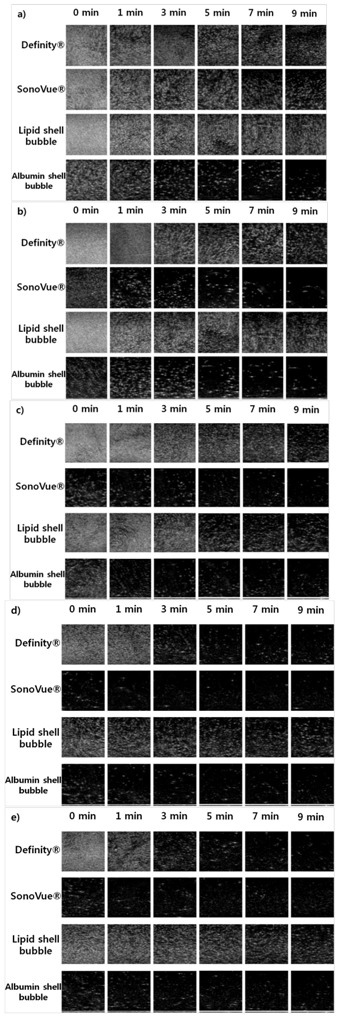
Ultrasound imaging comparison of two commercial UCAs and two synthesized UCAs over time (0, 1, 3, 5, 7, 9 min). (a) 3 days after synthesis. (b) 6 days after synthesis. (c) 9 days after synthesis. (d) 12 days after synthesis. (e) 15 days after synthesis. Ultrasound imaging results show that the synthesized microbubbles can be successfully used for imaging purposes. In particular, the synthesized lipid shell UCA shows almost equivalent imaging capabilities as Definity^®^. On the other hand, the synthesized albumin shell UCA seems to less effective in increasing ultrasound imaging contrast.
